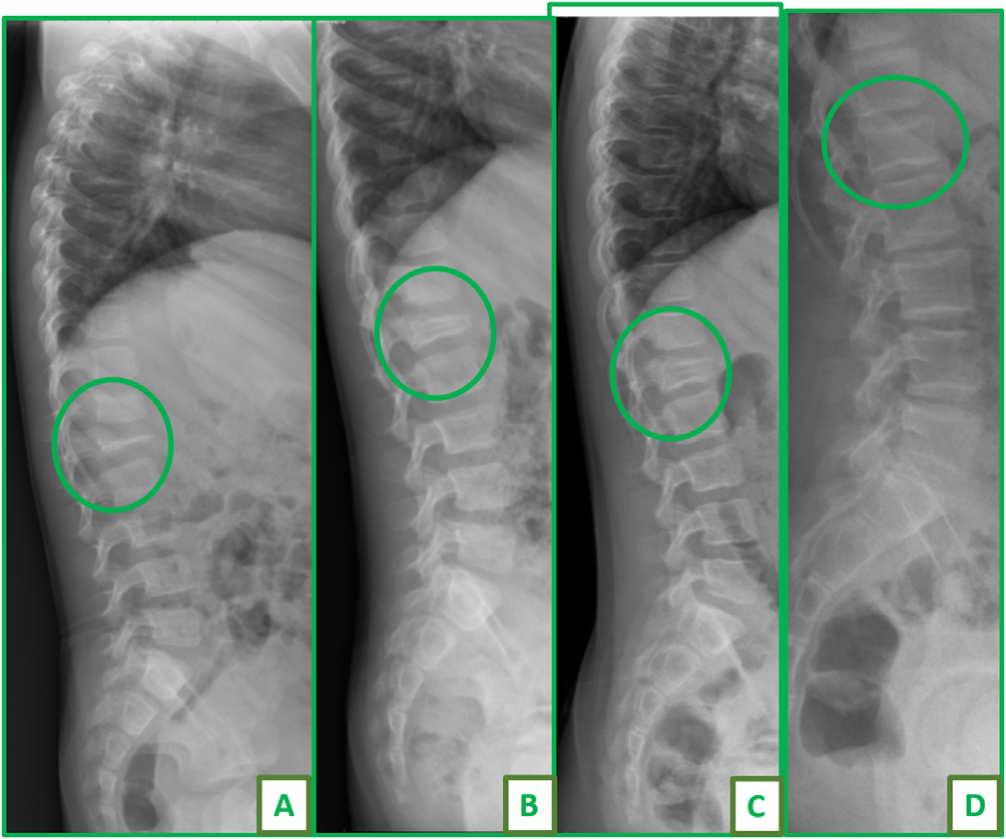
Plain radiograph of a skeletal lesion in the lumbar spine L1 of a 3-year-old boy managed conservatively. (A) Marked flattening of L1, vertebra plana. (B) Gradual remodeling and restoration of the height after 1-year follow-up. (C) Remodeling after 2 years. (D) Complete restoration of height after 5-year follow-up, child age 8 years.
